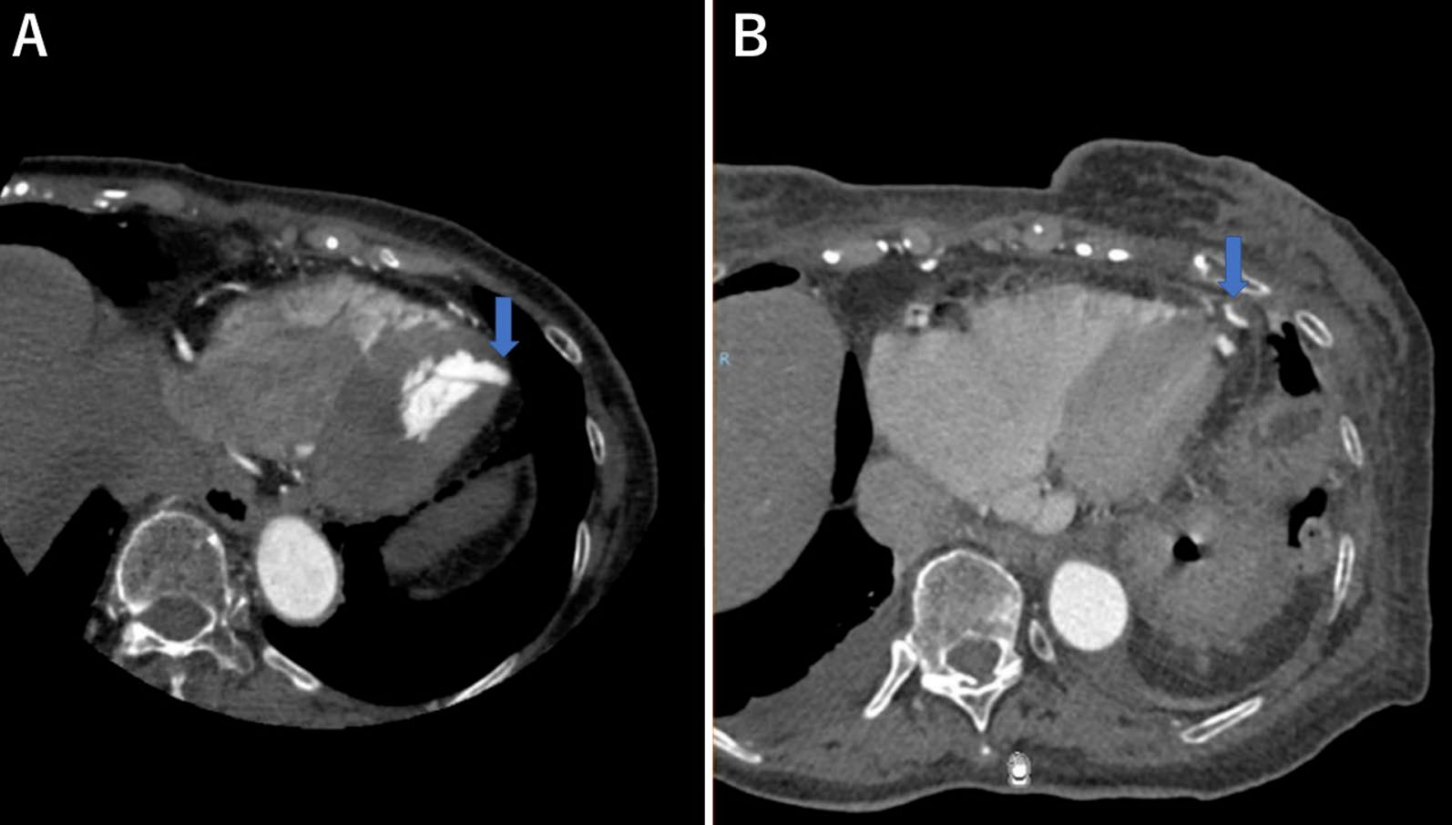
Pre- and postoperative CT scan images. a Preoperative CT scan images. Allows: Left ventricle aneurysm. b Postoperative CT scan images. *CT* computed tomography
